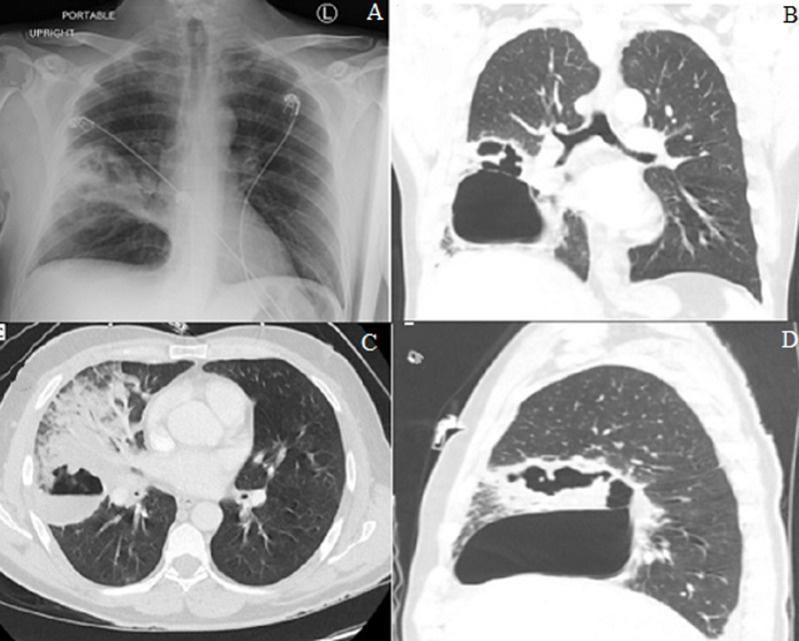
A) chest X-ray on admission showing focal infiltrate in the right midlung zone involving the minor fissure with multiple areas of lucencies suggestive of abscess formation; B) computed tomography (CT) axial view demonstrating dense consolidation of the right middle lobe (RML) and abscess with air-fluid level; C) coronal CT with revealing RML necrotizing pneumonia as well as thick wall cavity in the right lower lobe (RLL); D) C) sagittal view with RML and RLL necrotizing pneumonia and abscess formation
